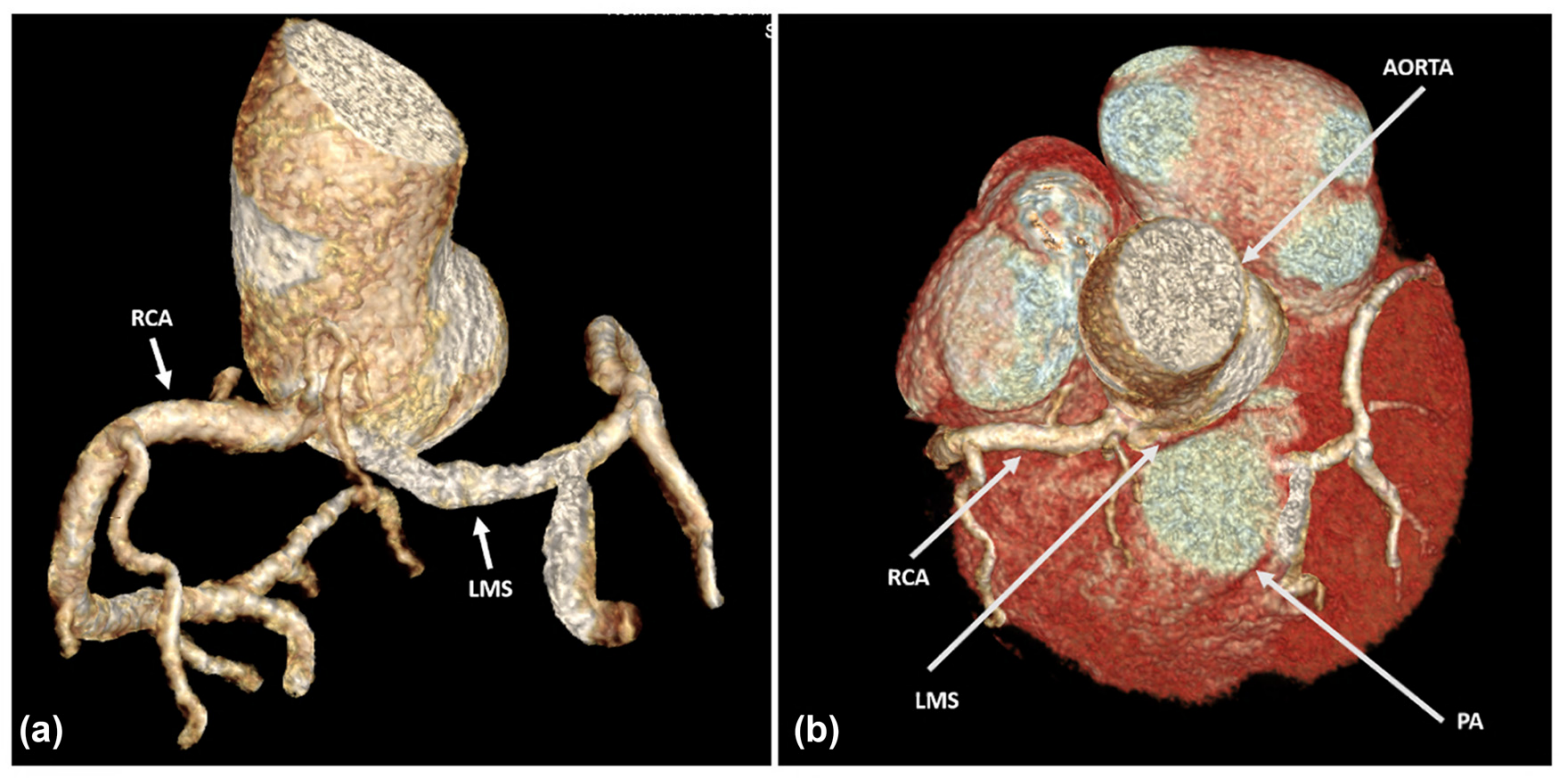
(a) Anterior view of a 3D rendered image of the CT coronary angiogram demonstrating anomalous origin of the LMS and right coronary artery (RCA) arising from the common origin of the right coronary cusp. (b) Aerial view depicting the LMS and RCA sharing a common origin right coronary cusp. The LMS can be seen to narrow as it traverses the inter-arterial between the aorta and PA.
